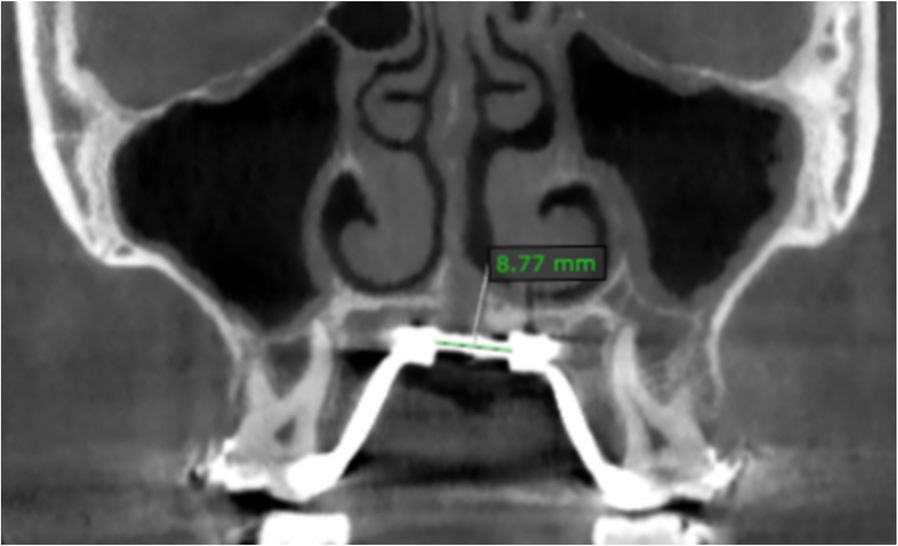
CBCT coronal section, showing the distance between the two halves of the MSE expansion jackscrew after expansion
